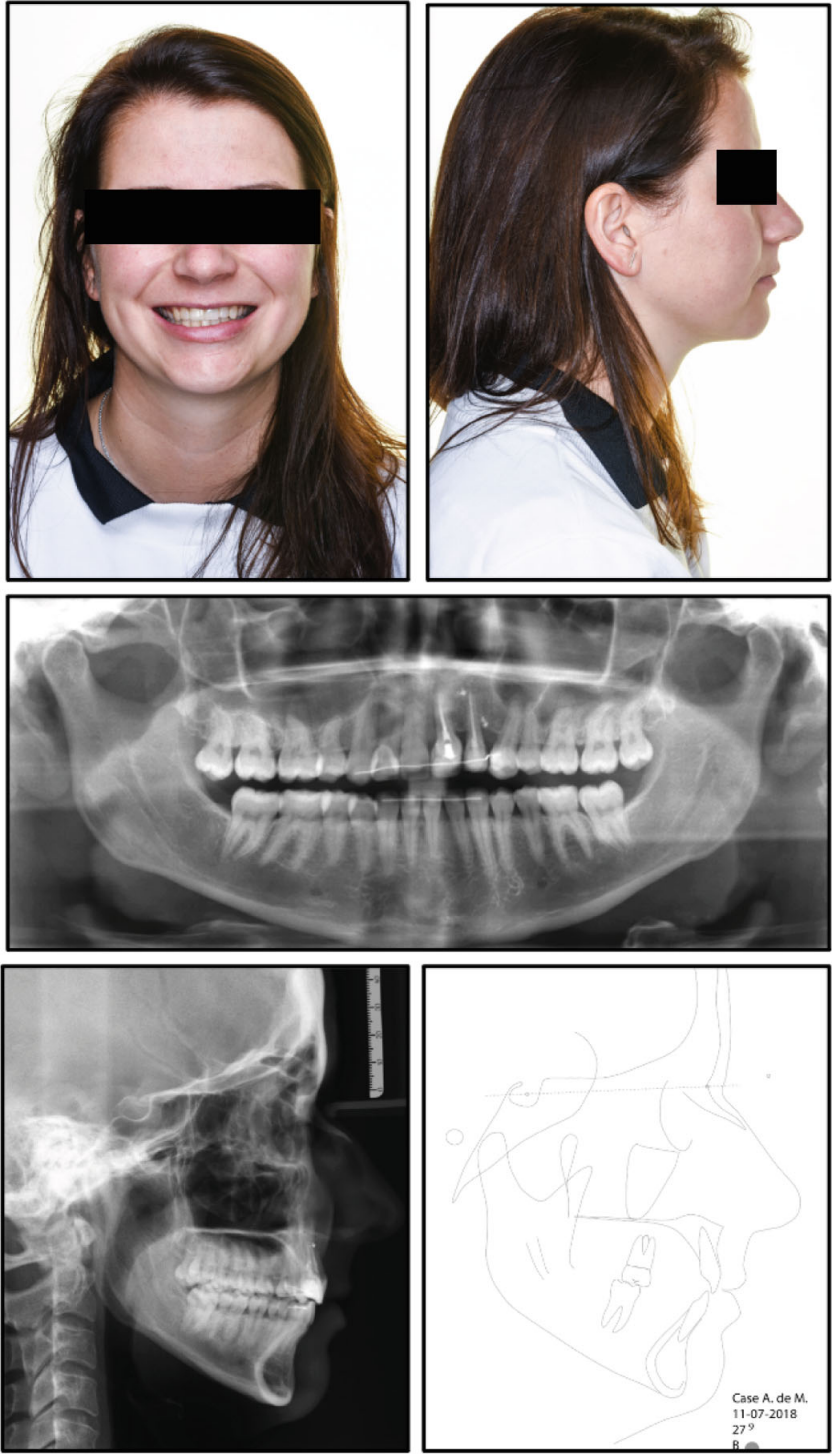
Facial analysis, panoramic and lateral radiographs, and cephalometry after treatment end.
